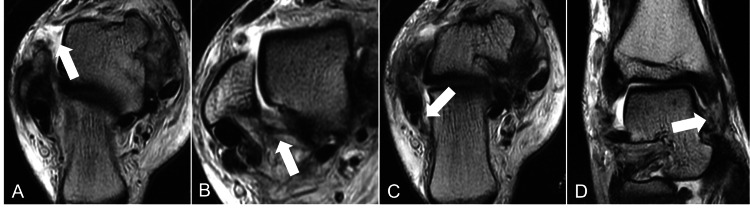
Postsurgery T2-weighted MRI of the patient's ankle. Axial T2-weighted MRI of the right ankle revealed tears in the anterior talofibular ligament (ATFL) (A), posterior talofibular ligament (PTFL) (B), and calcaneofibular ligament (CFL) (C) (arrow). The deltoid ligament (DL) (D) was indistinct in the deep layers, but the shallow layers remained (arrow). No cartilage damage to the talus was observed.
